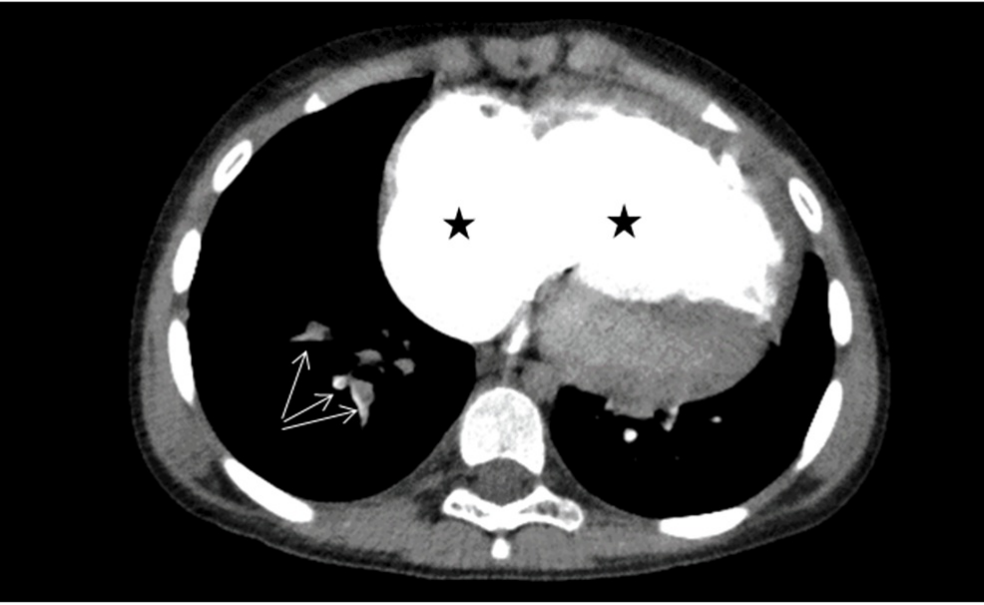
Chest CT angiography of the patient. Asterixis demonstrates enlargement of the right atrium and right ventricle secondary to right-sided heart strain because of pulmonary artery hypertension. Arrows point to filling defects (pulmonary emboli) in the segmental pulmonary arterial branches supplying the right lower lobe.
